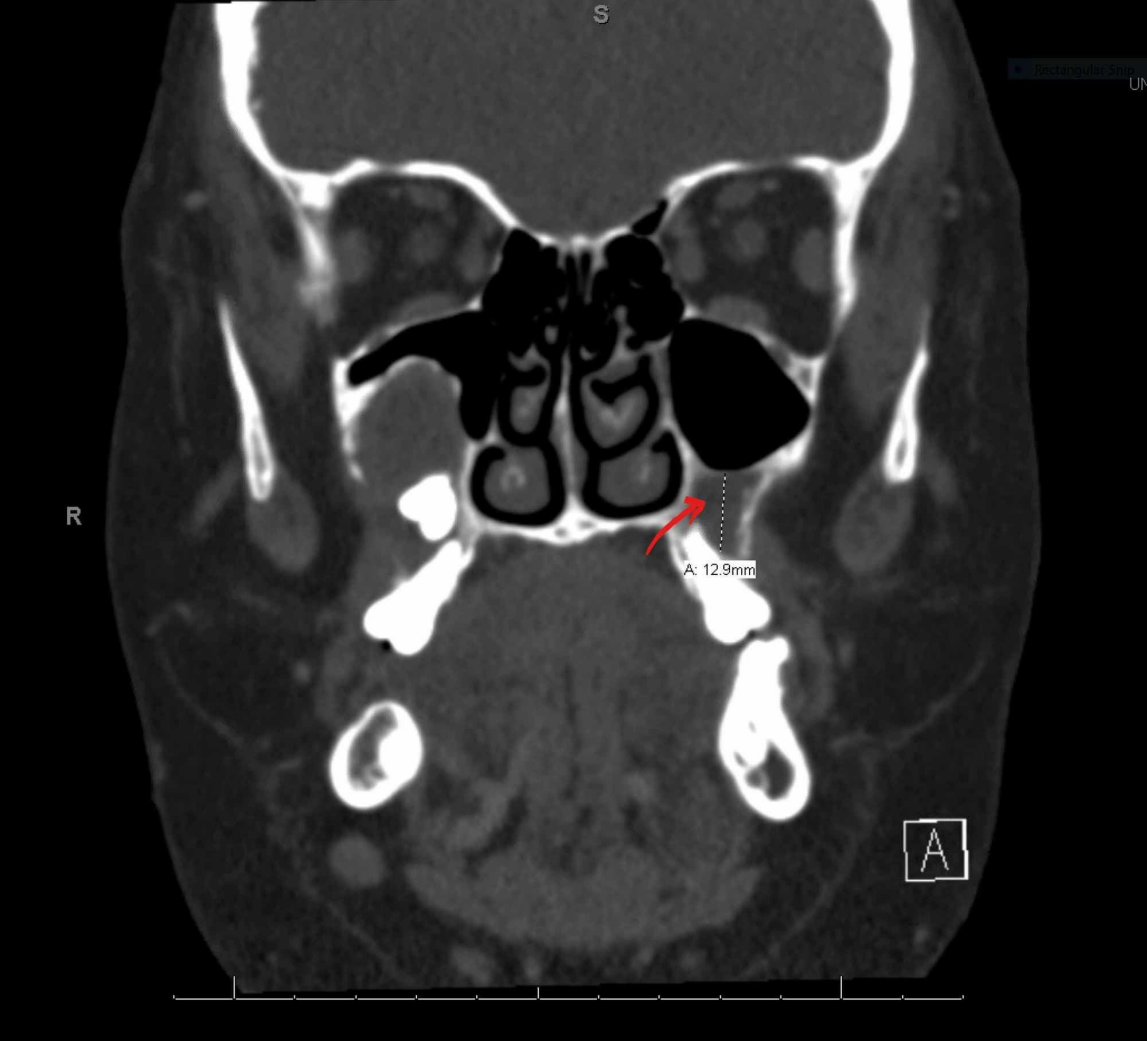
CT maxillofacial and neck showing left maxillary molar (unilocular) dentigerous cyst measuring 1.2 cm x 1 cm x 1 cm. The red arrow is indicating the left maxillary dentigerous cyst.
